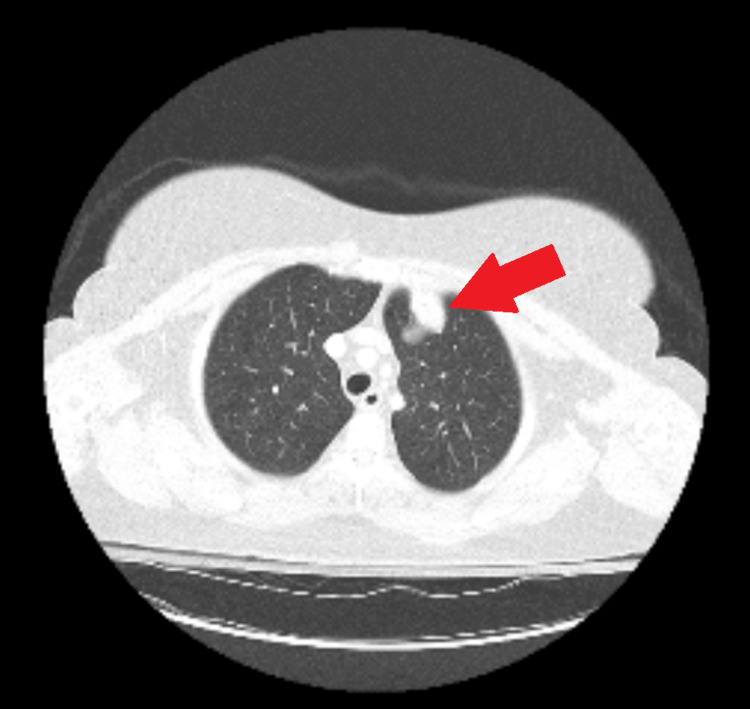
Computed tomography (CT) of the chest showing the patient’s left apical lung mass
